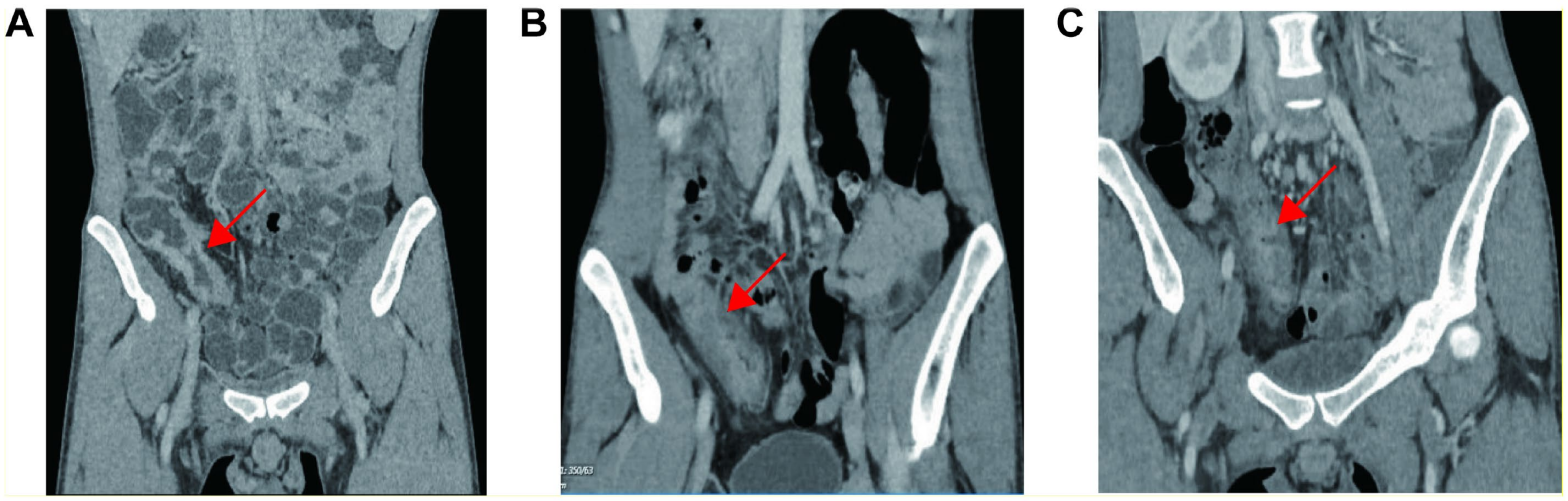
Serial radiologic assessment of ileocecal involvement. (A) Contrast-enhanced CT at baseline demonstrated marked bowel wall thickening and luminal narrowing (arrows). (B) After infliximab induction (week 14) residual stenosis persisted (arrows). (C) Following therapeutic drug monitoring–guided infliximab dose intensification (every 4 weeks × 2), no further radiologic improvement was evident.
